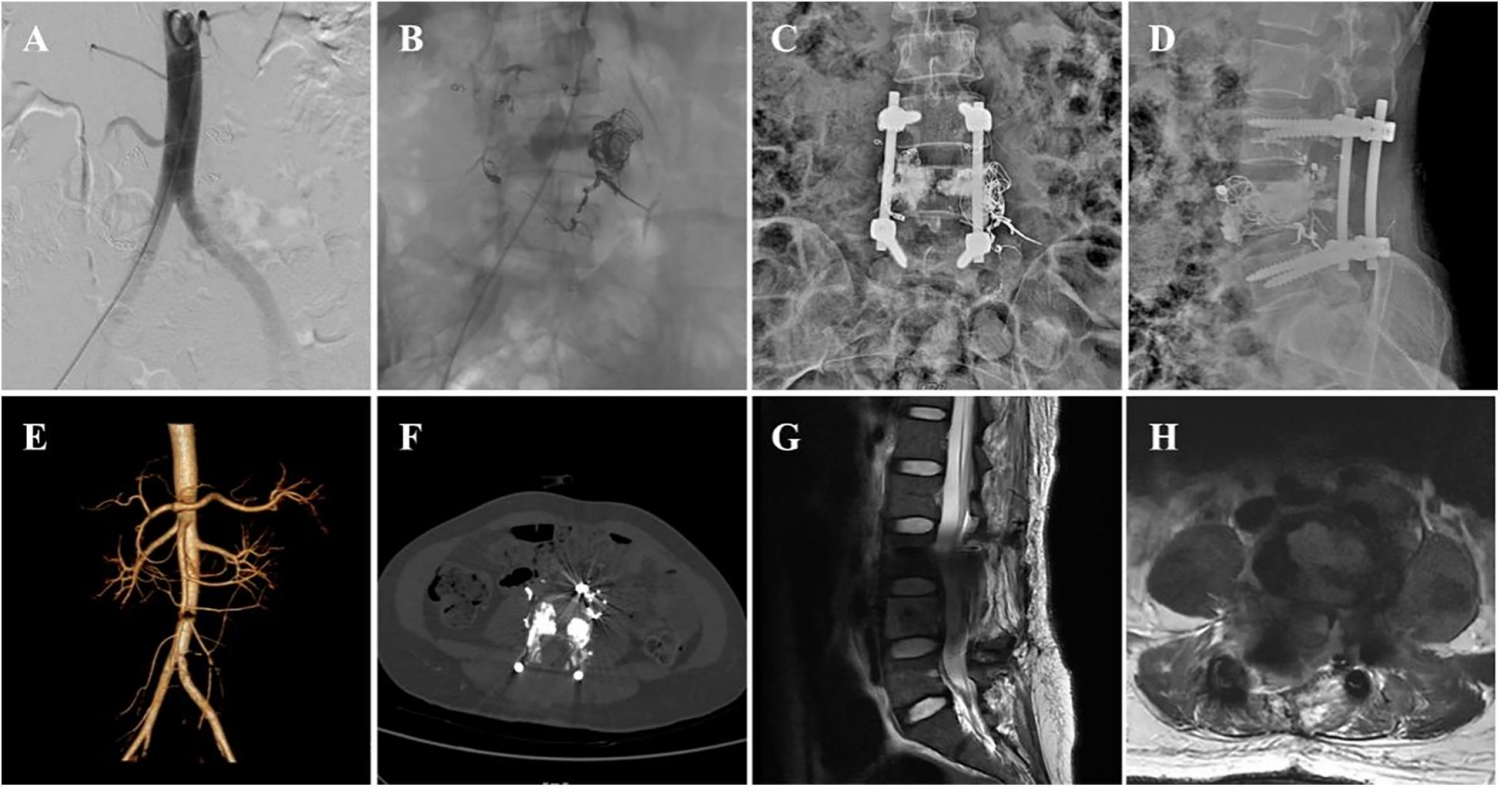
Postoperative imaging data of the patient. (A,B) postoperative DSA images, (C,D) postoperative x-ray anteroposterior and lateral views, (E,F) postoperative CTA images, and (G,H) postoperative MRI images.
